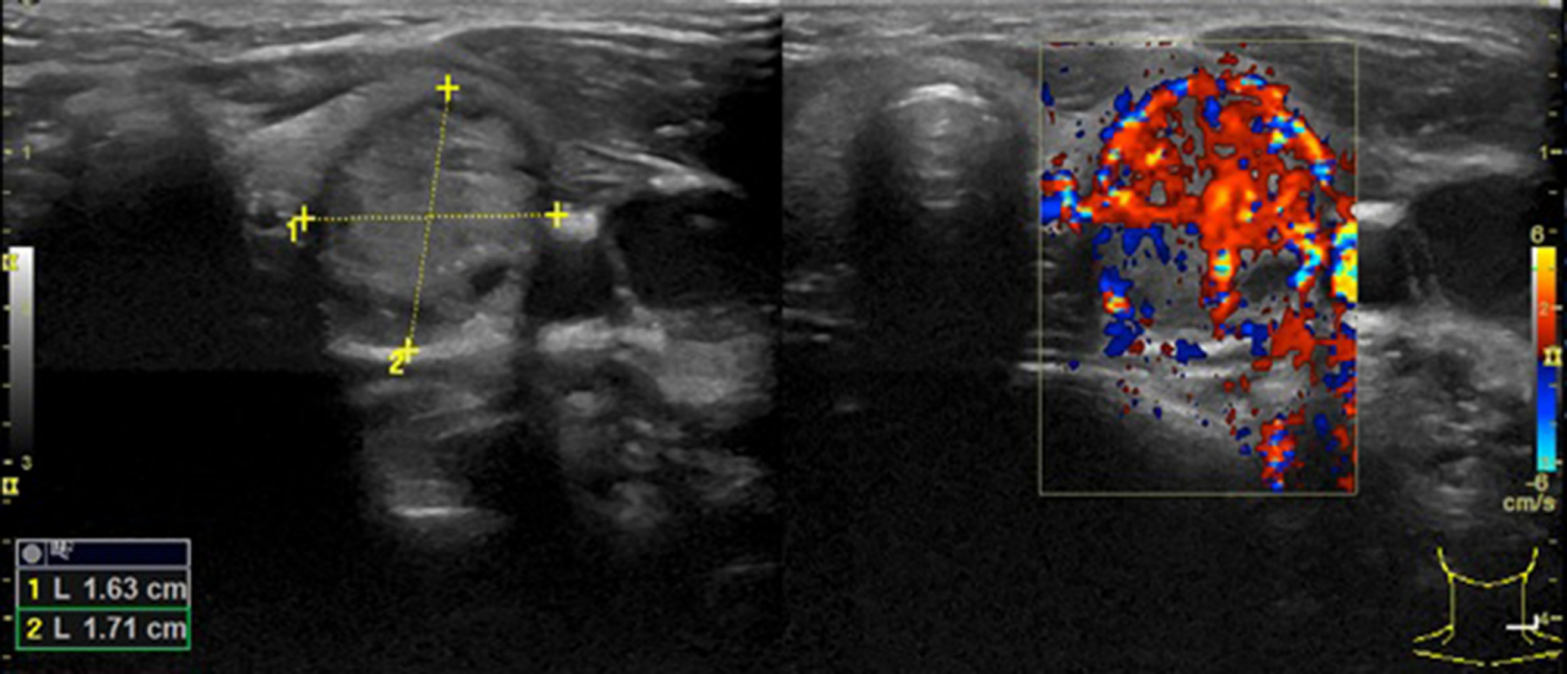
The first thyroid ultrasound examination showing a solitary thyroid nodule with an LD of 17 mm in the left lobe.
